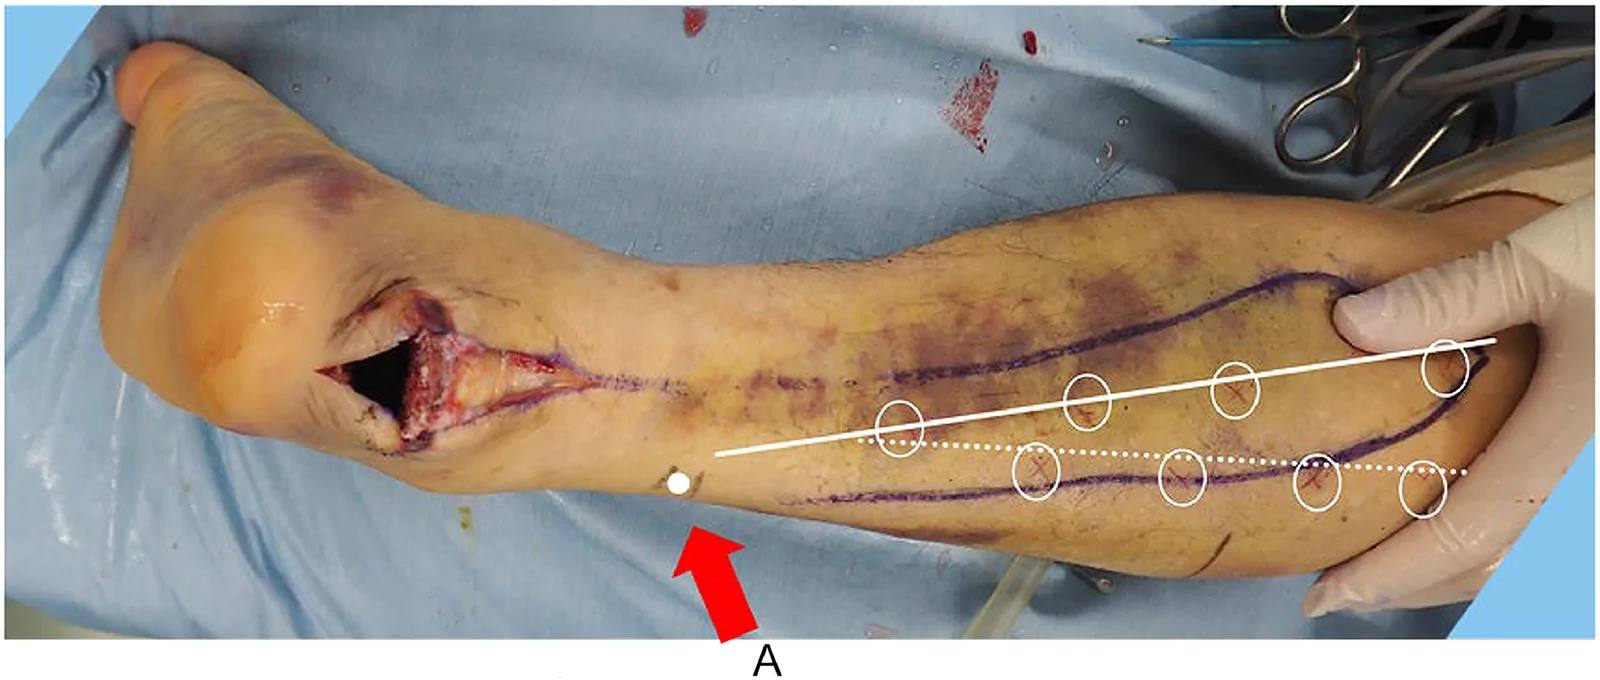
Flap design and marking with ultrasonography. The peroneal perforator was identified via ultrasonography and marked as Point A. The medial sural nerve and median superficial sural artery were marked (white circles on solid line a). The flap axis was drawn by connecting them to Point A (solid line a). The lateral sural nerve was also marked (dashed line b).
